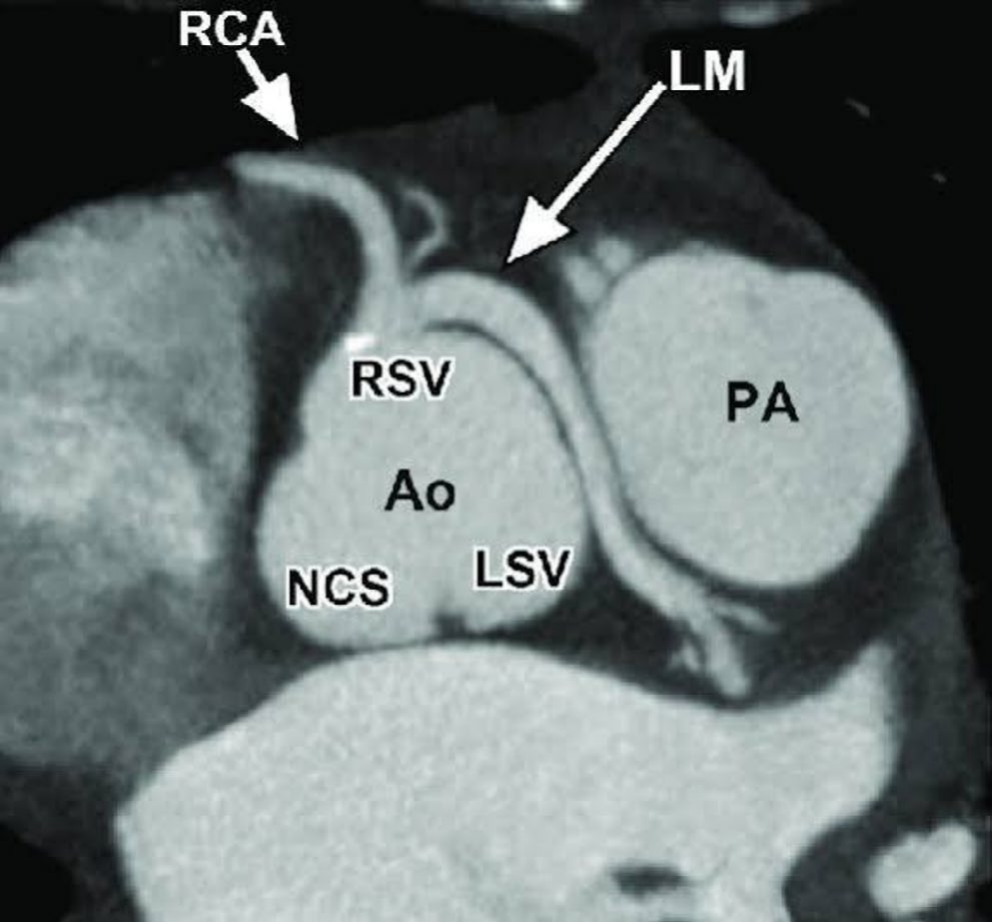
Coronary computed tomography angiogram showing the left main coronary artery (LM) arising anomalously from the right sinus of Valsalva (RSV, arrow), and coursing between the aorta (Ao) and pulmonary artery (PA). The right coronary artery (RCA) and left coronary sinus (LSV) are labeled.
